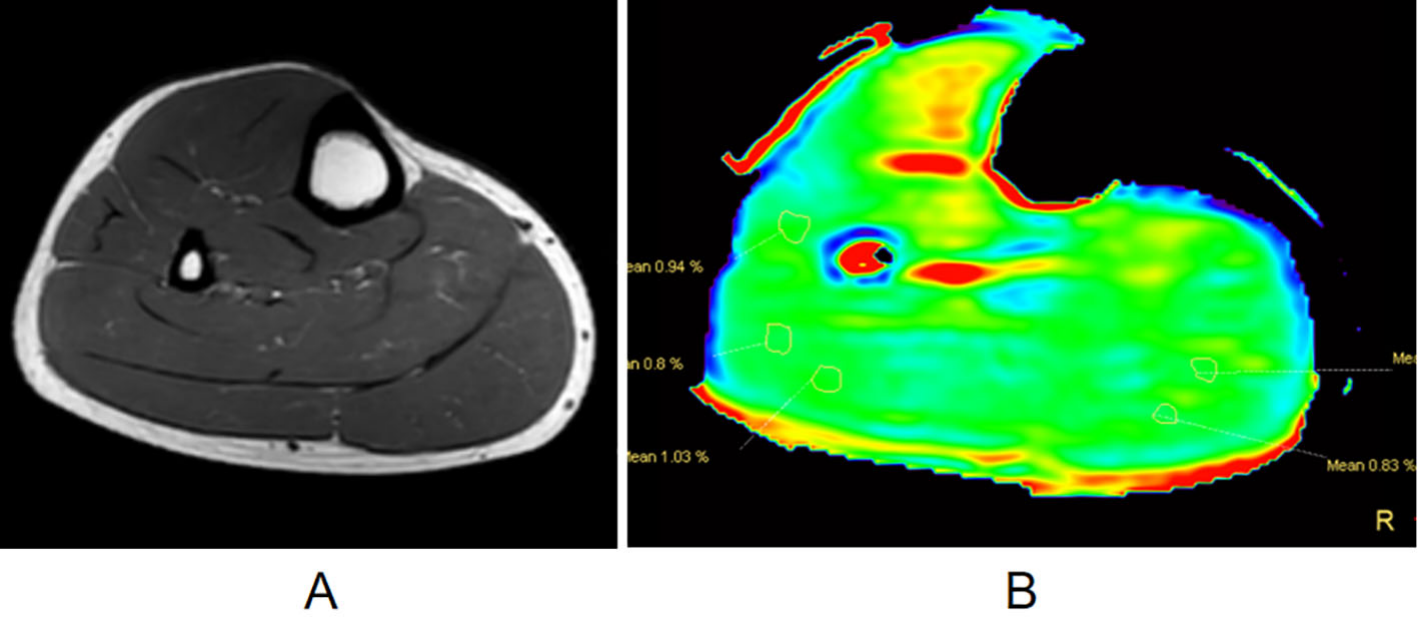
ROI selection method. The tumor was in the tibia. We selected the ROI in the tibia on the APT image. (A) Axial T2WI; and (B) APT image. APT, Amide proton transfer; ROI, region of interest; T2WI, T2-weighted imaging.
